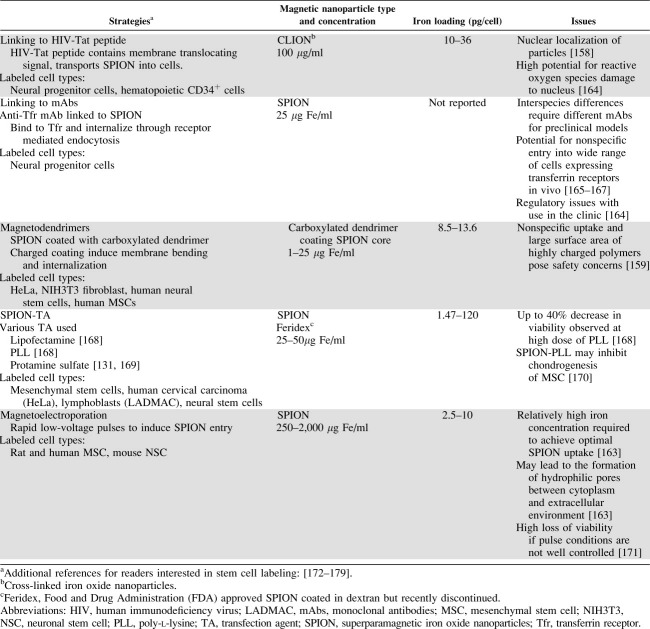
#### Use of Other Nanolabels Can Improve MRI Detection of SCs.
